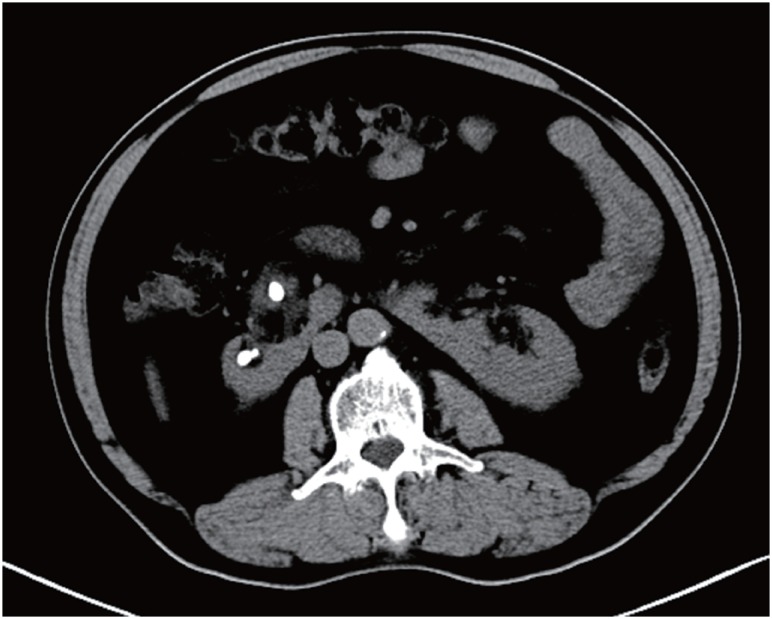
CT scan of renal stones in a horseshoe kidney.
